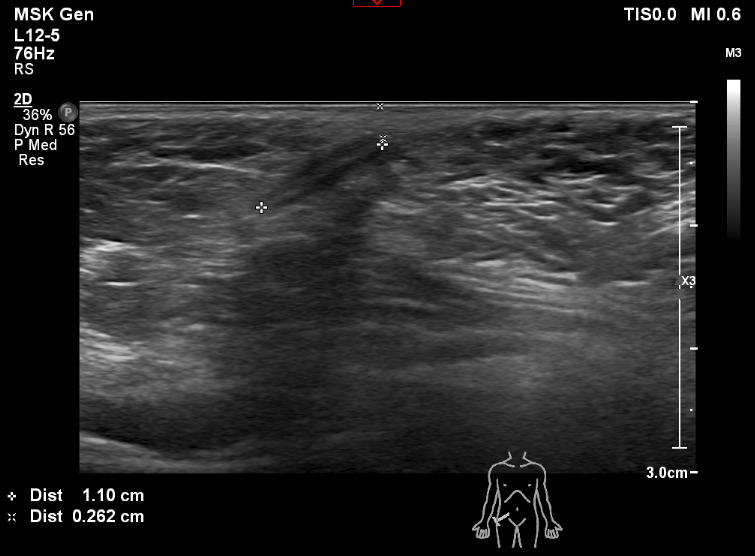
Transverse ultrasound image of the foreign body identified in the inguinal region.
